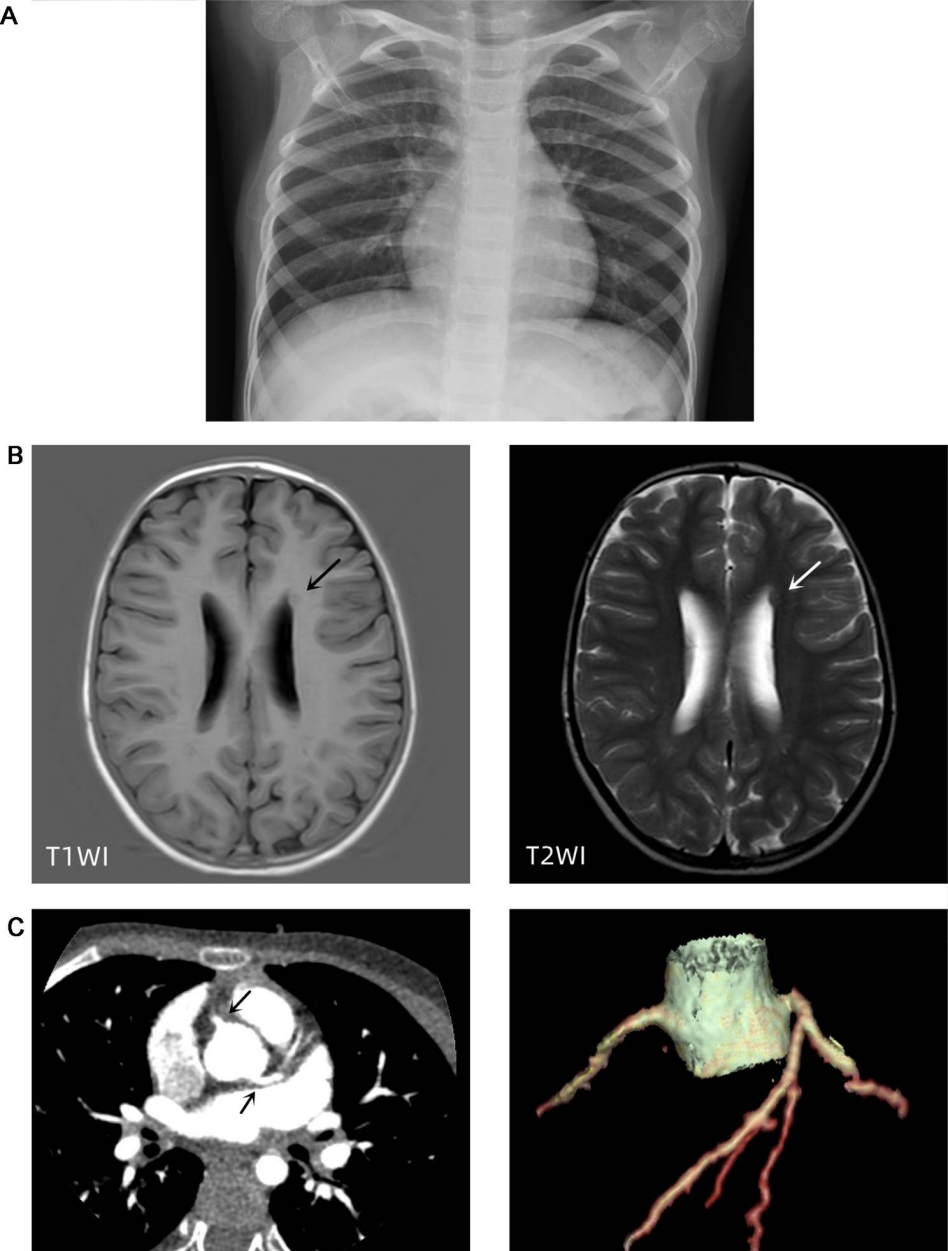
A The chest X-ray was normal. B Cerebral MRI scan displayed abnormal nodular signals adjacent to the left anterior horn of the lateral ventricle, which the arrows point to. C Coronary artery CT angiography showed no abnormality in the origin and course of the bilateral coronary arteries. The arrows point to the location where the left and right coronary arteries originate
